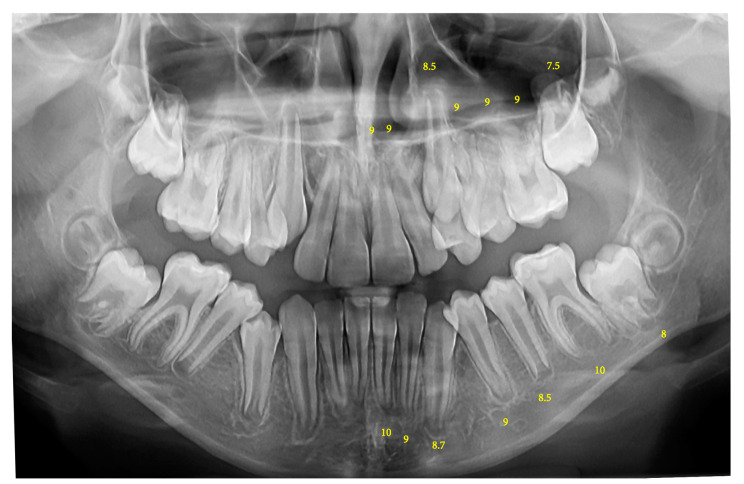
Scoring teeth on a panoramic radiograph using the Nolla method.
